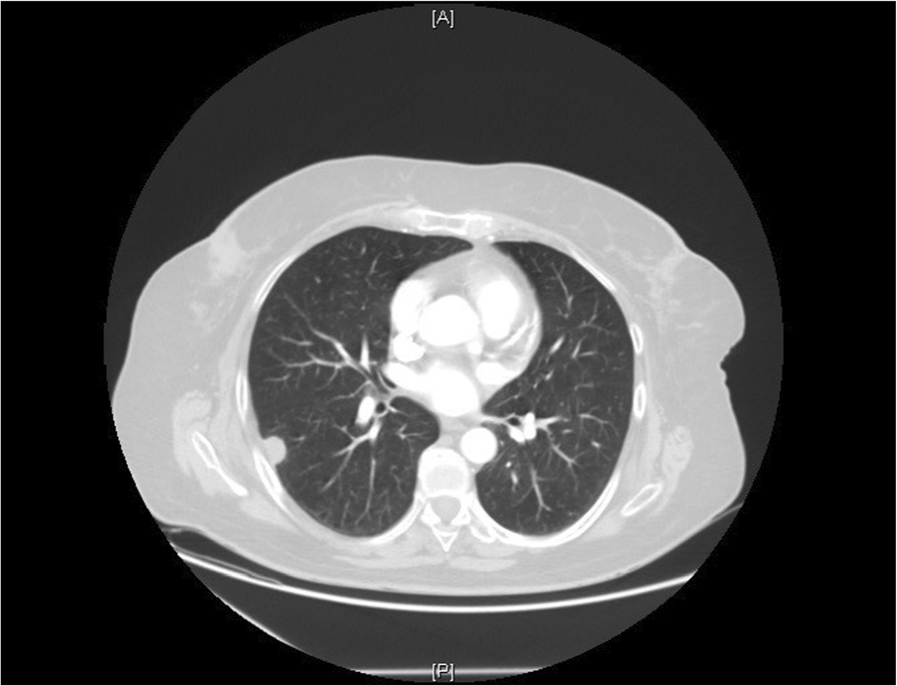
CT scan showing pulmonary right lower lobe nodule
